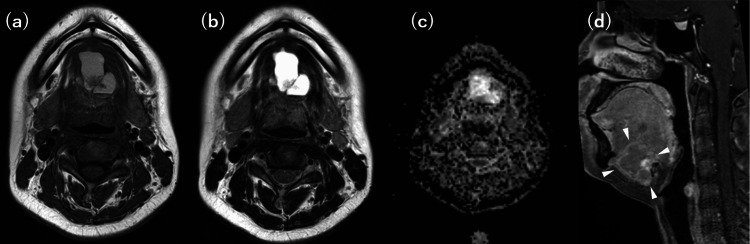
Preoperative contrast-enhanced magnetic resonance imaging (MRI) scans (a) Axial T1-weighted image. (b) Axial T2-weighted image. (c) Apparent diffusion coefficient (ADC) map. (d) Contrast-enhanced sagittal T1-weighted image. The mass demonstrates slightly high signal intensity on T1-weighted images and high signal intensity on T2-weighted images, consistent with a hemorrhagic cyst. The solid components of the mass show contrast enhancement and mild reduction in ADC values, raising suspicion for malignancy.
